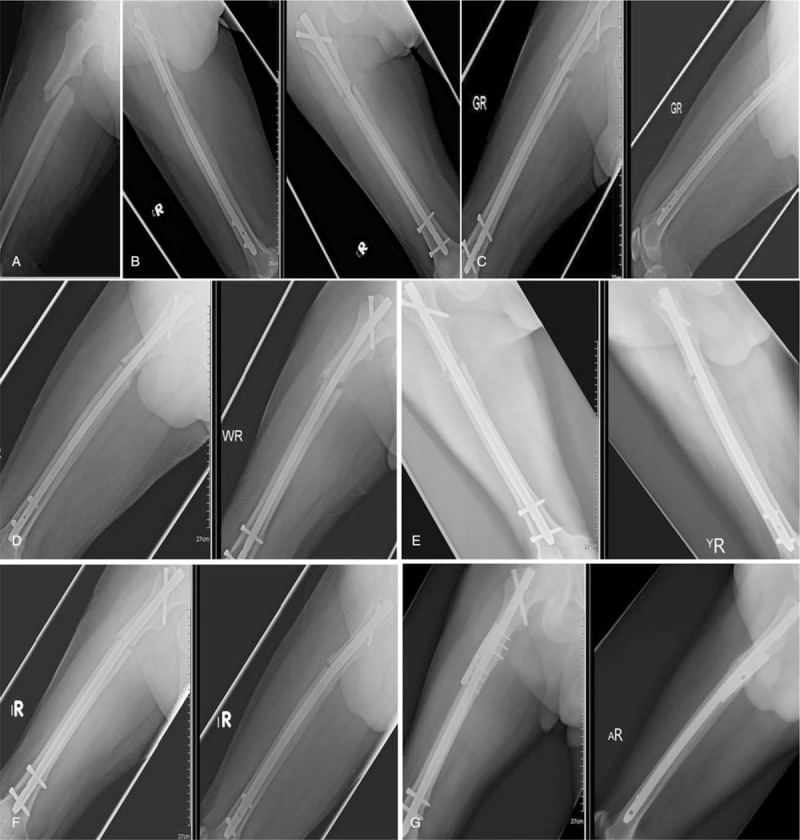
51 y/o obese man suffered from traffic accident. (A) Immediate X-ray after trauma showed right femur shaft fracture at proximal to third junction; (B) postoperative 1 day X-ray; (C) postoperative 3 months X-ray; (D) postoperative 6 months X-ray showed oligotrophic nonunion of the fracture site; (E) post-second-operation 1 day X-ray showed thicker nail and chipped bone graft over fracture site; (F) post-second-operation 4 months X-ray showed nail broke at the fracture site; (G) post-third-operation 8 months X-ray showed solid union of the fracture site.
